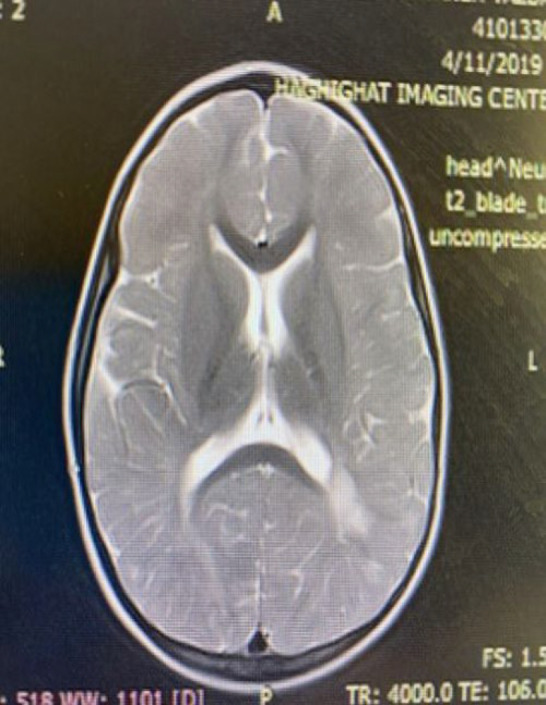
Male sibling axial T2 weighted brain MRI image shows periventricular gliosis
